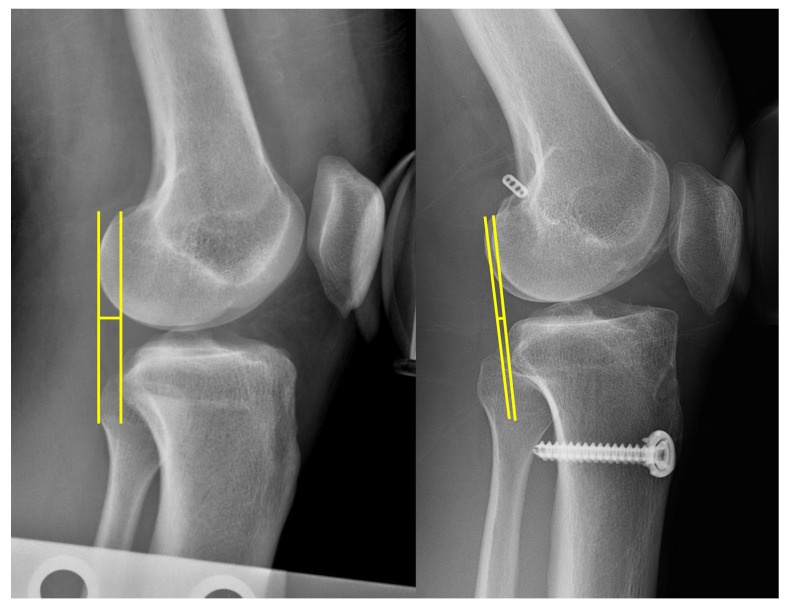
Measurement of preoperative and postoperative anterior tibial translation on anterior stress radiography. (yellow lines) Two perpendicular lines were drawn tangentially to the most posterior contour of the femoral condyle and tibial plateau. Anterior tibial translation was defined as the distance between the two perpendicular lines.
